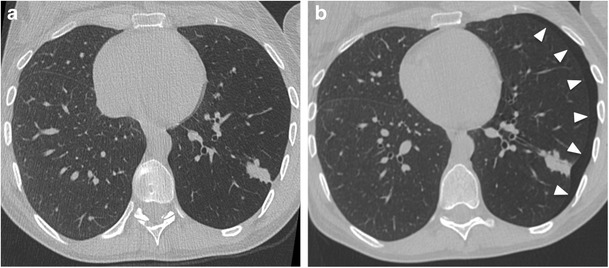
CT-guided chest biopsy of solitary pulmonary nodule in the left lower lobe. (a) Axial CT image before the procedure showing the subpleural nodule. (b) Post-procedural axial CT image showing small PNX (arrows) as a complication of transthoracic biopsy
